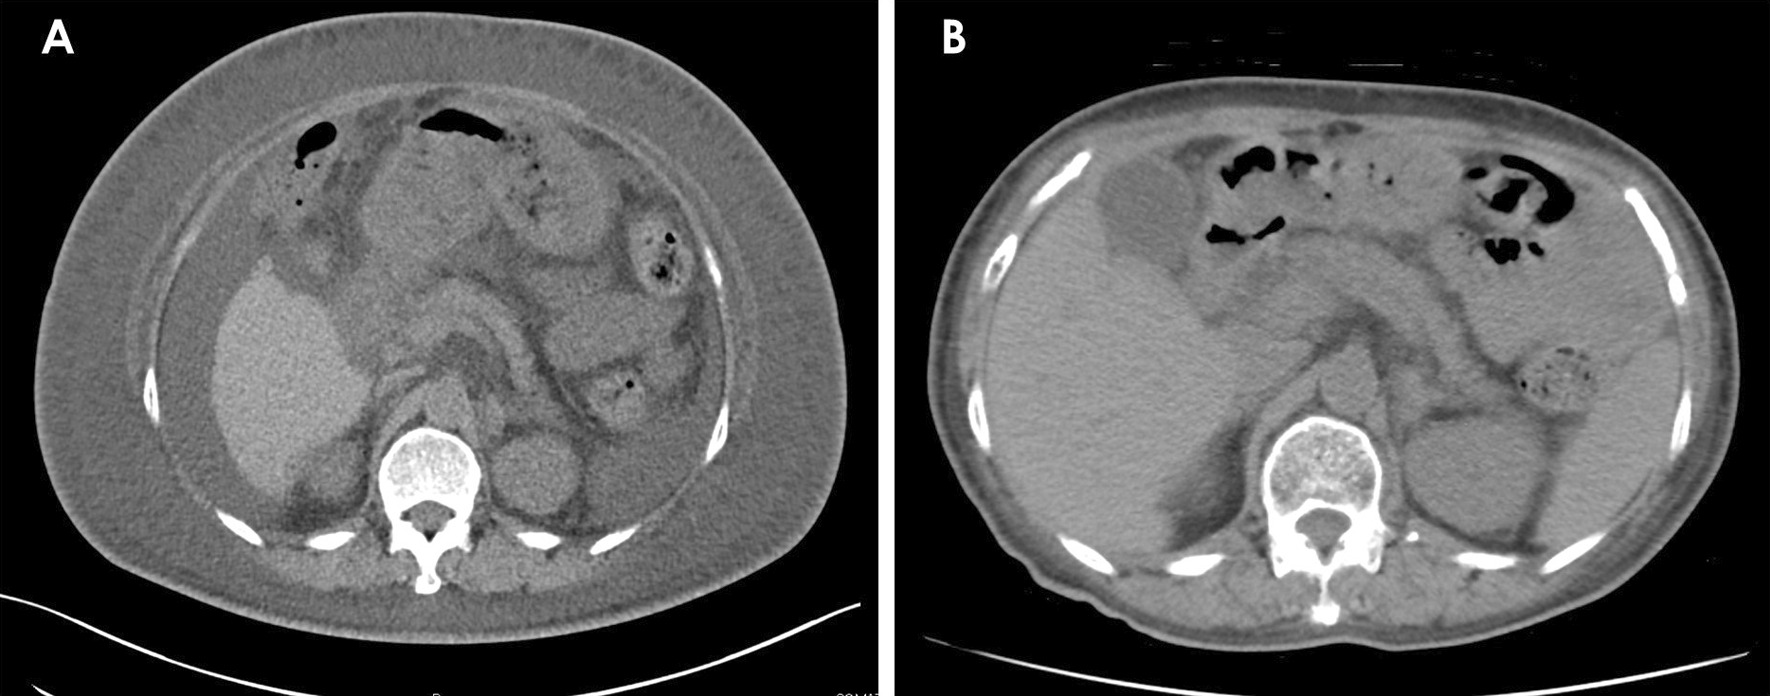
A Computed tomography showed subcutaneous edema and ascites. B After *Helicobacter pylori* eradication, subcutaneous edema and ascites were improved
